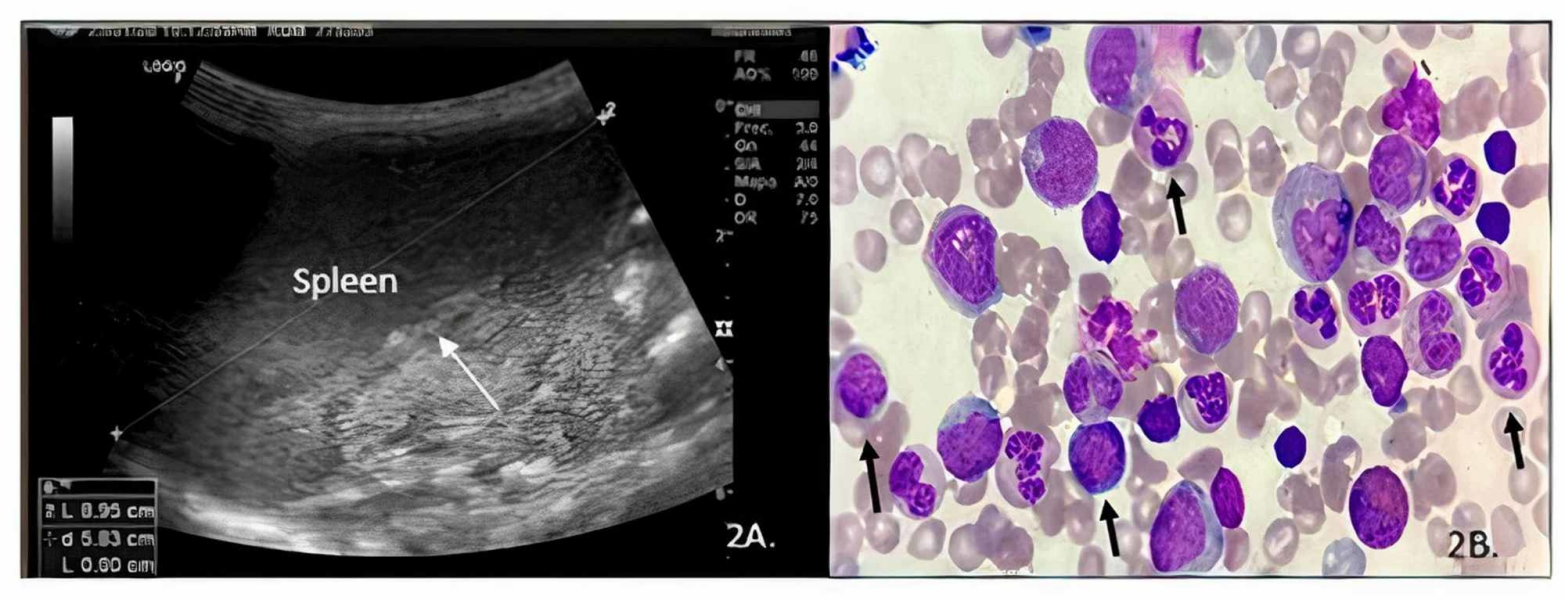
A. Ultrasound of the abdomen that showed splenomegaly; B. Myelogram was hypocellular with mainly granulocytic and erythroid hematopoiesis
